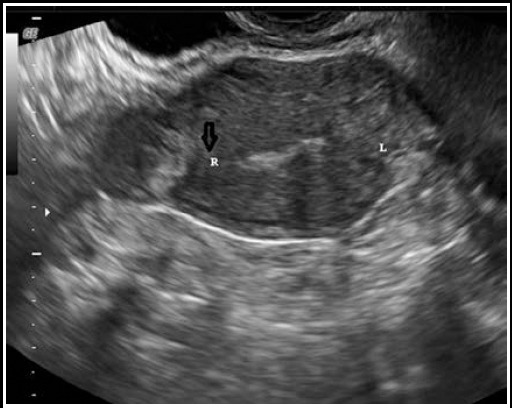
Coronal view of the fundus of the uterus on transvaginal ultrasound showed a mass measuring 2 cm in the right uterine horn and the interstitial line sign (black arrow).
